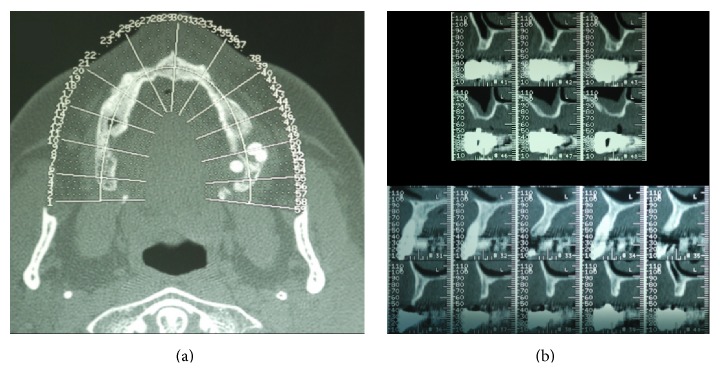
The CT dental scan confirms the clinical view showing atrophic maxillary ridge with important horizontal bone defect. (a) It is possible to underline all the atrophies of the upper maxilla; (b) a particular of the axial section of the area involved in the grafting procedures.
